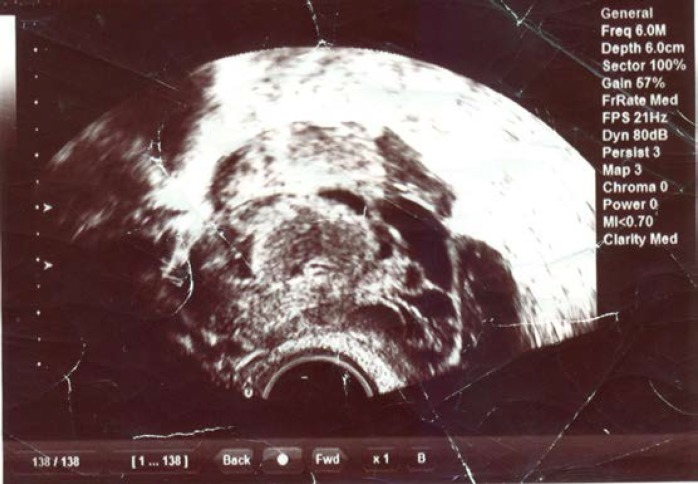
Transvaginal ultrasonography image
